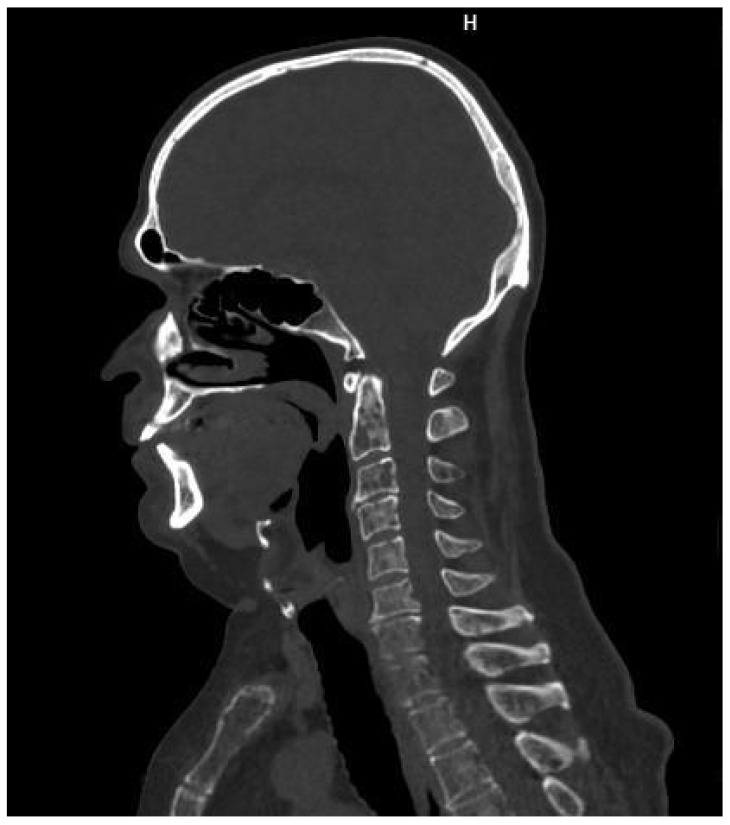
A 63-year-old man with lytic lesions on the cervical spine, shown in whole-body low-dose computed tomography. Source: Radiological Diagnostics Center, The National Institute of Medicine of the Ministry of Interior and Administration, Warsaw, Poland.
